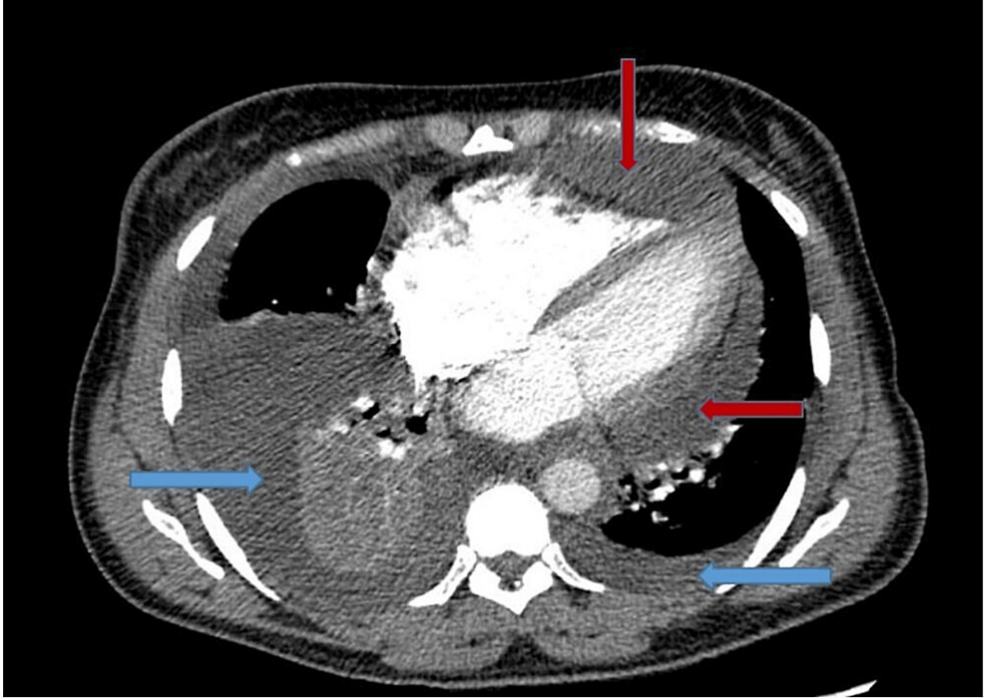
CT of chest showing a large pericardial effusion (red arrows), a large right-sided pleural effusion, and a small left-sided pleural effusion (blue arrows)
